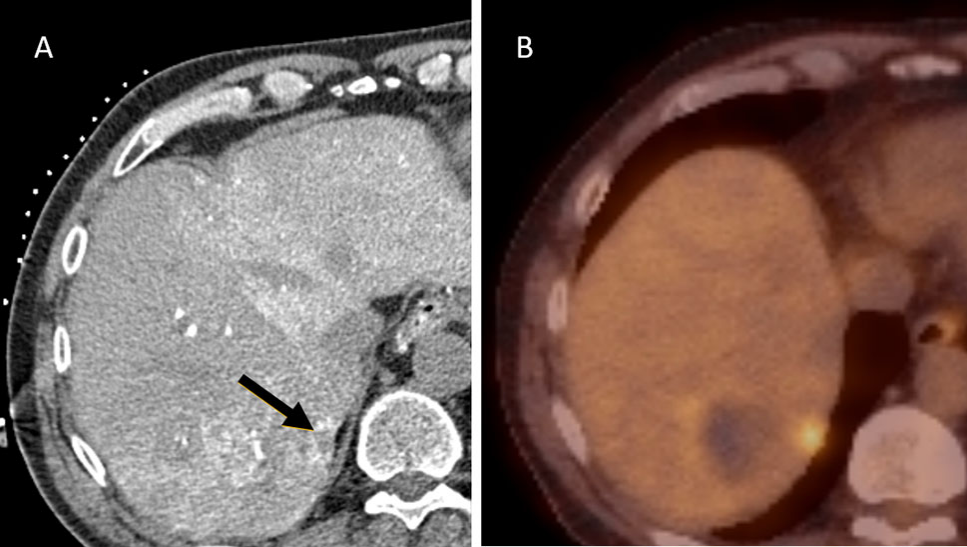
A intraprocedural transcatheter CTHA. B: follow-up 18F-FDG PET-CT 2,5 months after CTHA-guided ablation. Retrospectively, an enhancing ring lesion was identified in segment VII on the CTHA (arrowheads), which had not been noticed during the microwave ablation
